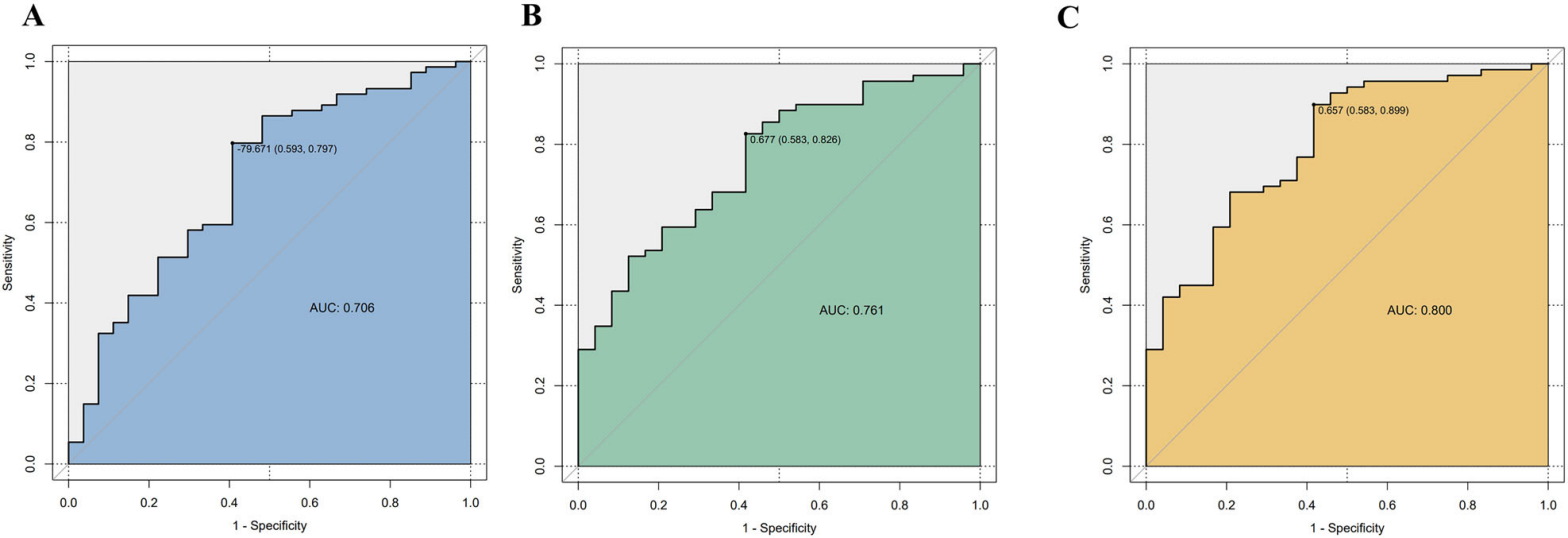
Diagnostic accuracy of FAI in the diagnosis and activity of TA. A Receiver operating characteristic curve of the FAI in activity of TA. B Model 1: aortic wall thickness and standardized CT value of aortic wall and number of affected branches; C FAI combined with Model 1. AUC, area under the curve
